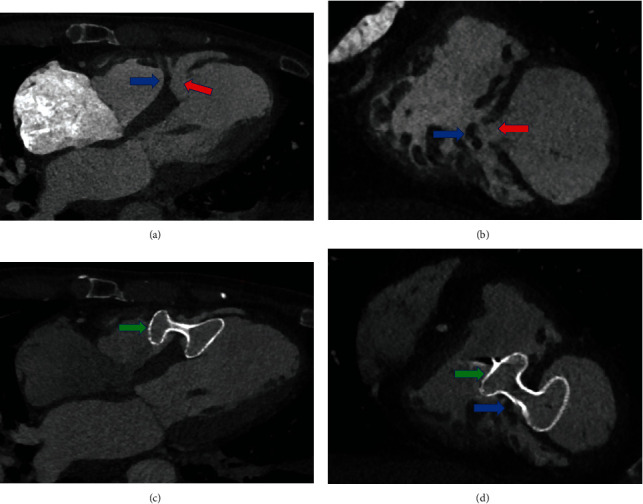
(a) Preoperative horizontal plane imaging by cardiac CTA. (b) preoperative coronal plane imaging by cardiac CTA, showing that the right ventricular surface defect has the moderator band barrier. (c) Postoperative horizontal plane imaging by cardiac CTA. (d) Postoperative coronal plane imaging by cardiac CTA, showing that the occluder waist diameter is severely compressed due to the moderator band barrier. The red arrow in the figure indicates the VSR position, the blue arrow indicates the adjustment band, and the green arrow indicates the occluder.
